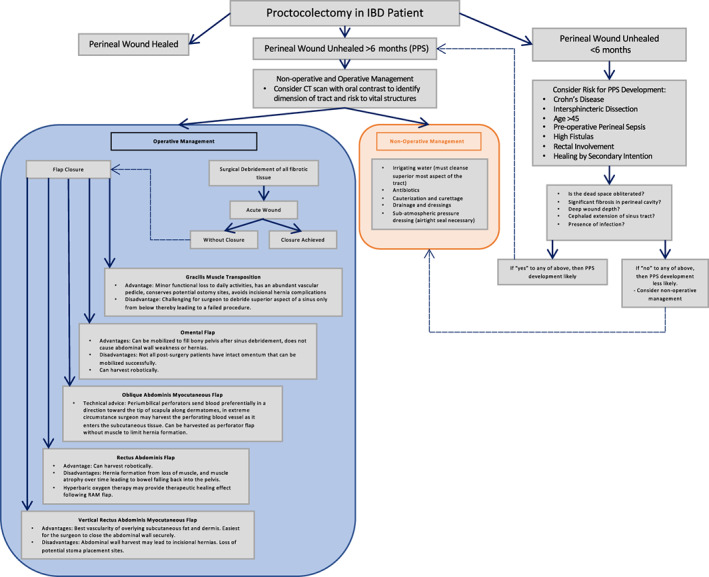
Algorithm for the diagnosis and management of persistent perineal sinus (PPS). CT, computed tomography; IBD, inflammatory bowel disease.
